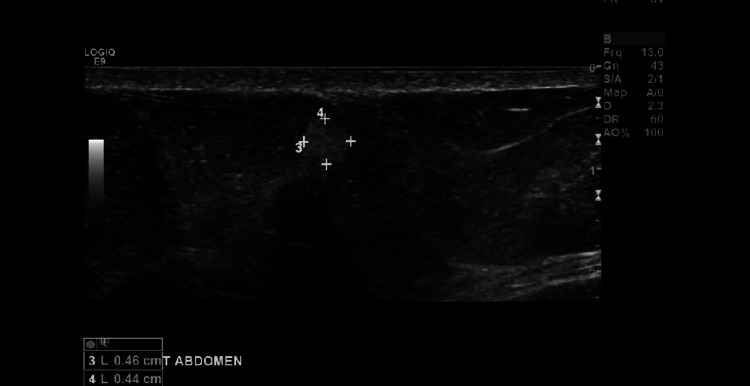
Ultrasound image of a 0.46 x 0.44 x 0.35 cm right abdominal soft tissue mass
